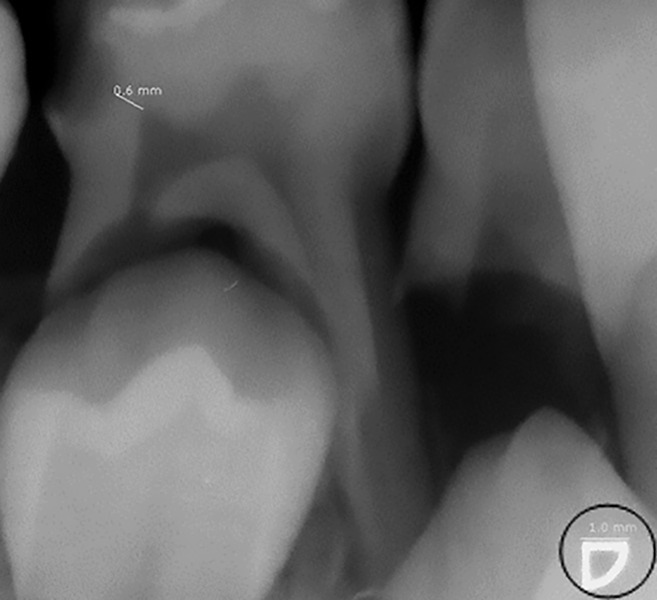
Radiograph showing the measurement of the RDT on a lower right primary first molar after software calibration (0.6 mm). The triangle circled in black in the bottom of the radiograph was used as a reference
